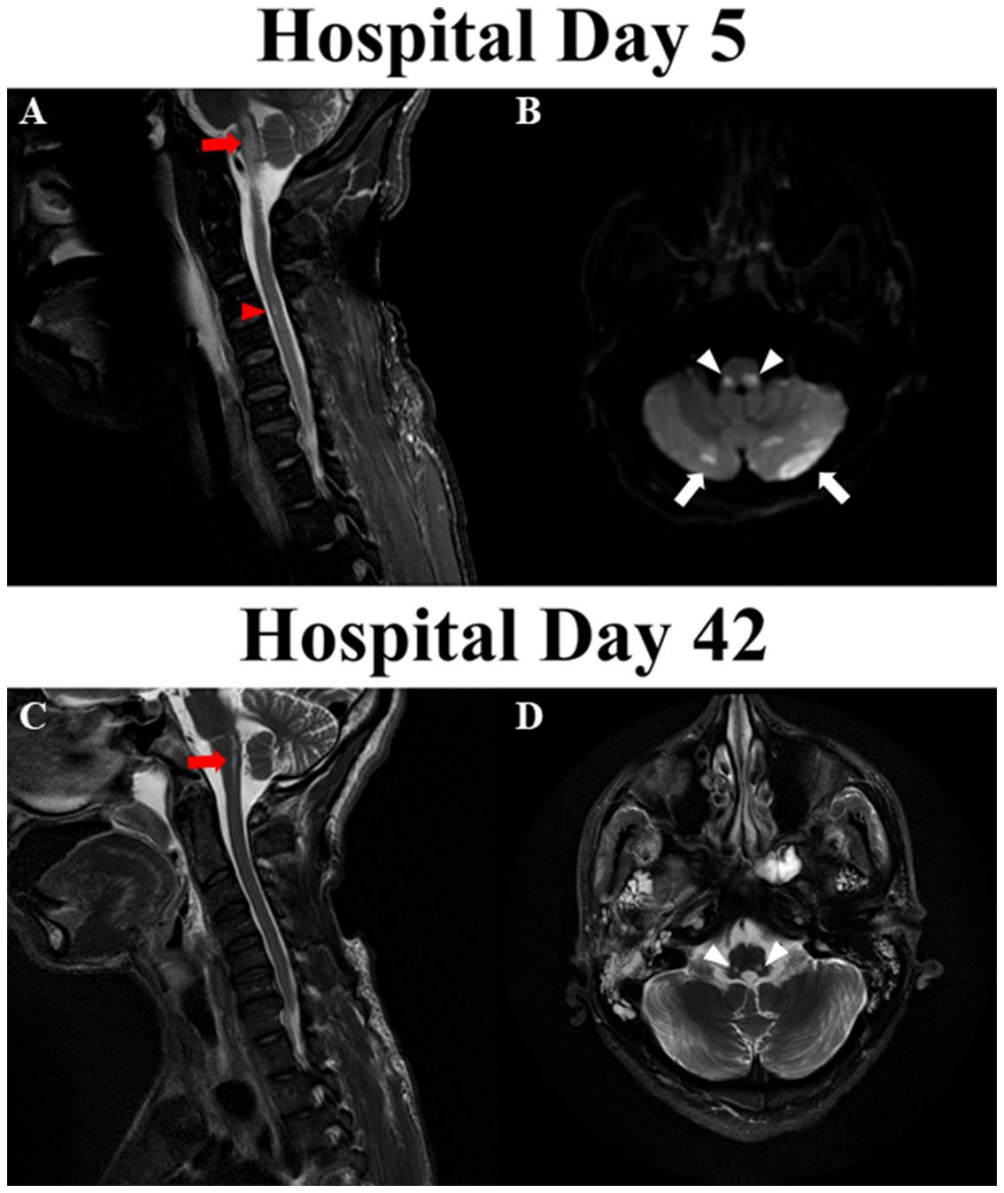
Magnetic resonance imaging of brain and cervical cord. (A) Fluid-attenuated inversion recovery (FLAIR) abnormalities in medulla oblongata (red arrows) and ventral aspect of C3-C4 cervical cord (red arrowheads) on hospital day 5. (B) Diffusion restriction in dorsolateral medulla (white arrowheads) and bilateral cerebellar hemispheres (white arrows) on hospital day 5. (C) Improvement in the FLAIR signal in the medulla oblongata (red arrow) and resolution of FLAIR abnormality in cervical cord on hospital day 42. (D) Remnant T2 signal abnormalities in the regions bilateral nucleus tracti solitarii on hospital day 42.
